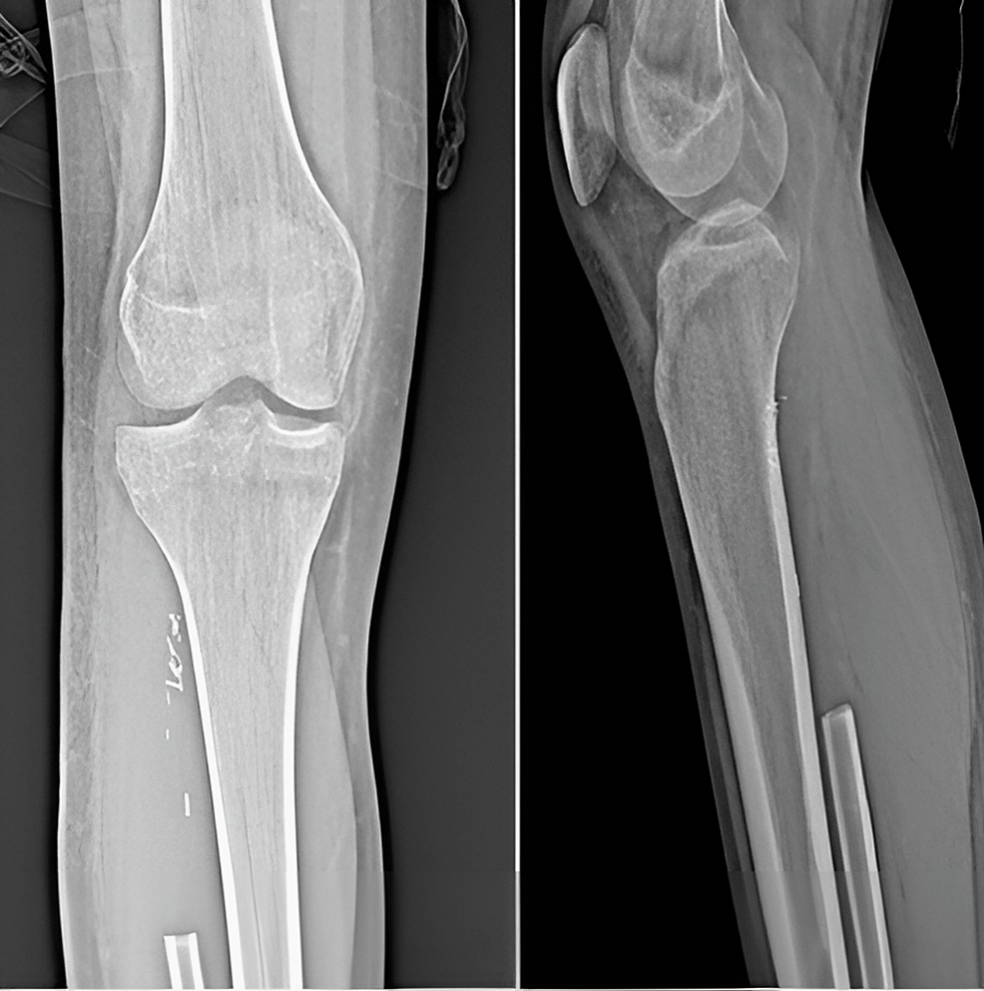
Radiograph of the patient at the one-month postoperative follow-up
